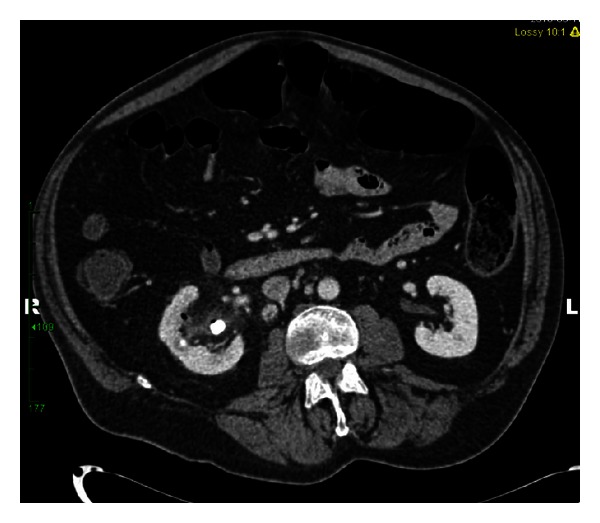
CT abdomen with contrast demonstrates right nephrolithiasis, stranding of the renal pelvis, and gas within the right collecting system.
